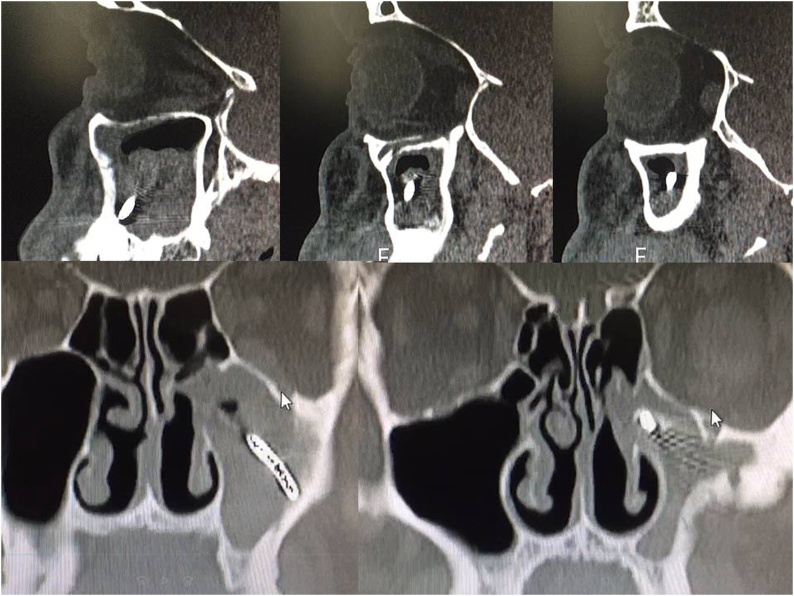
Sinus computed tomography (CT) executed without contrast evidenced the “presence of a metal foreign body with thread-like shape located in the region of the left maxillary sinus, which determined intense inflammatory reaction of the sinonasal mucosa interesting the same maxillary sinus”. Additionally, “the ipsilateral anterior ethmoidal air cells were partially involved”. Radiological findings of minor importance were the presence of left concha bullosa and nasal septum deviation, while the other nasal sinuses were within normal limits.
